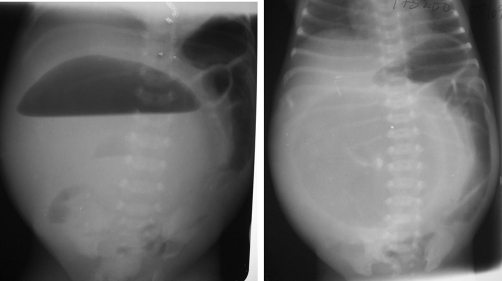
Plain abdominal radiographs (erect and supine) of an 11-hour-old boy with giant meconium cyst. Note the giant central mass with air-fluid level (left image), the peripherally displaced bowel loops with no remarkable distension, the elevated diaphragms to the level of 6th rib posteriorly and the splaying of the ribs.
